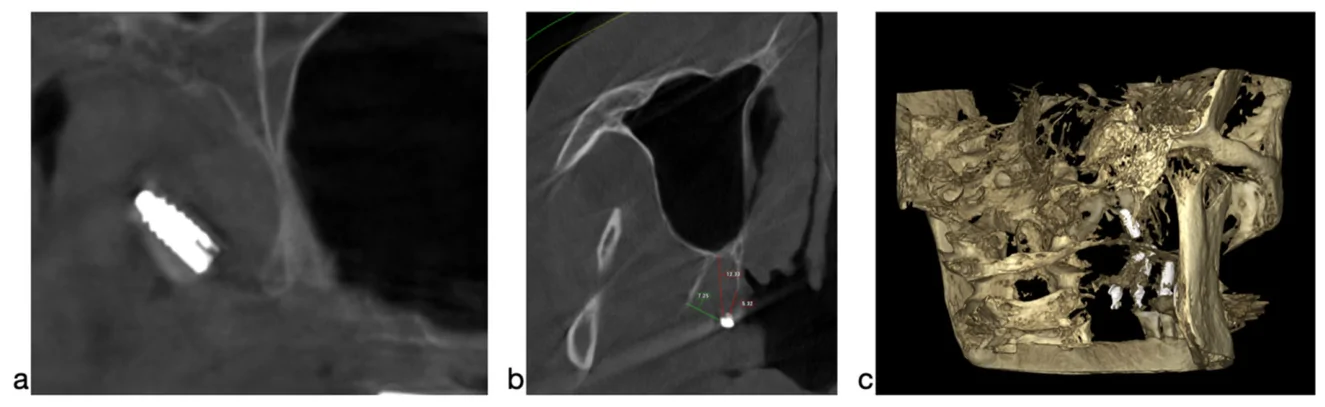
CBCT localization of the displaced implant. (a) Sagittal view showing the displacement. (b) Axial view showing the implant medial to the lateral pterygoid plate, anterior to the pterygoid process, within the pterygopalatine fossa. (c) Three-dimensional volume rendering of the displaced implant.
